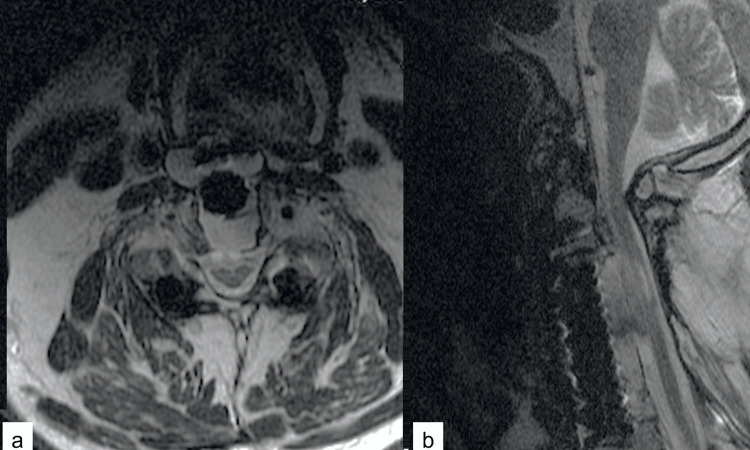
Postoperative MRI Postoperative axial (a) and sagittal (b) T2-weighted images show a decrease in the cerebrospinal fluid collection, and an increase in spinal cord diameter, seen better on the axial image
